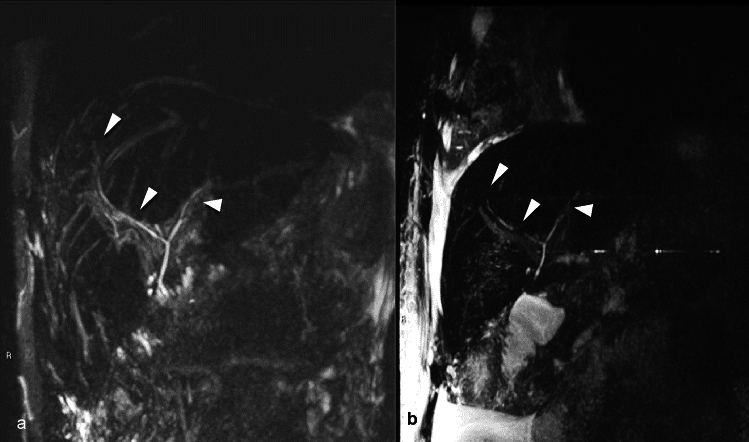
A 24-year-old male with orthotopic liver transplant for autoimmune hepatitis, after two previously failed transplants due to chronic rejection and complications from hepatic arterial thrombosis, presents with increased bilirubin levels of 30 mg/dL. MRI with Magnetic resonance cholangiopancreatography performed two months post-transplantation shows no dilation in the central bile ducts (arrowheads, a). The peripheral bile ducts are poorly visualized, raising concerns for ductopenia. A follow-up imaging six months later demonstrates multiple levels of bile duct obliteration (arrowheads, b) and non-visualization of the peripheral ducts. The persistent hyperbilirubinemia and bile duct loss, in the absence of hepatic arterial abnormalities, raised concerns for chronic rejection, which was confirmed by liver biopsy
